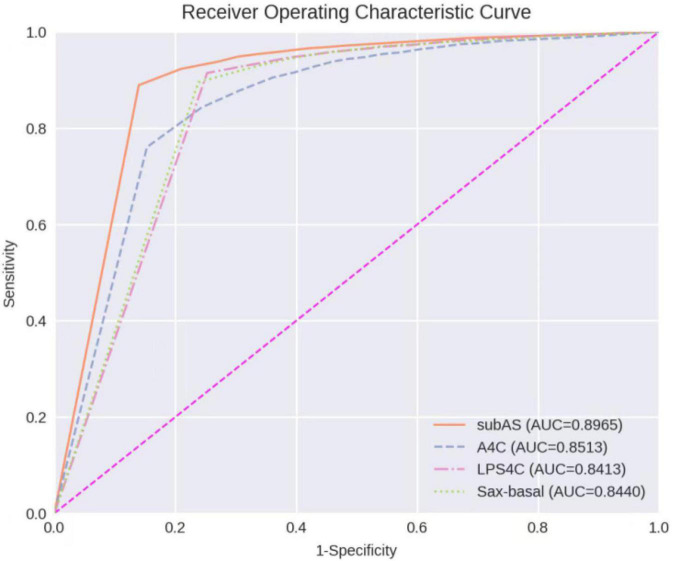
Receiver operating characteristic curves of ASD detection on four target echocardiographic views.
